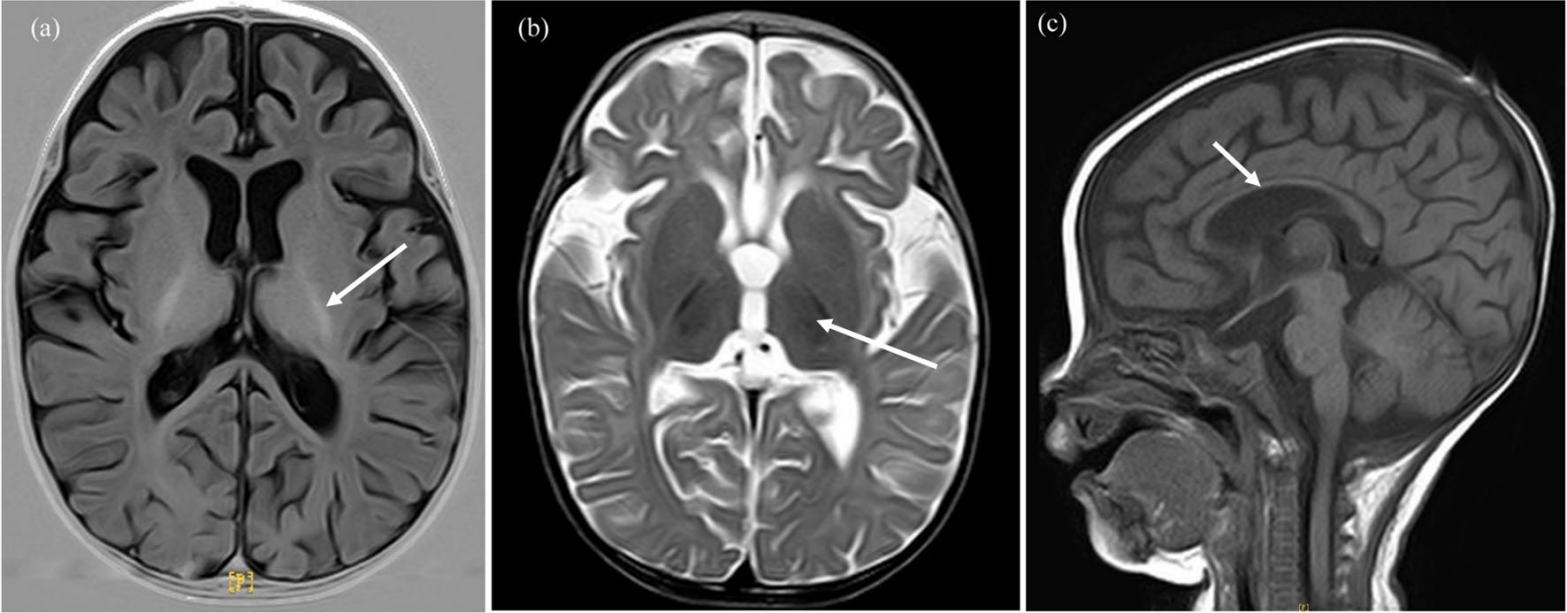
Brain magnetic resonance images (MRI) of the patient at seven months. T1‐weighted images (a and c) and T2‐weighted images (b). a The posterior limb of the internal capsule partly displays high intensity, suggesting myelination (arrows). b The posterior limb of the internal capsule displays low intensity, suggesting delayed myelination (arrows). c The gyri became bigger, and the sulci became deeper, while the hypoplastic corpus callosum showed hypointensity, suggesting progressive myelination (arrows)
